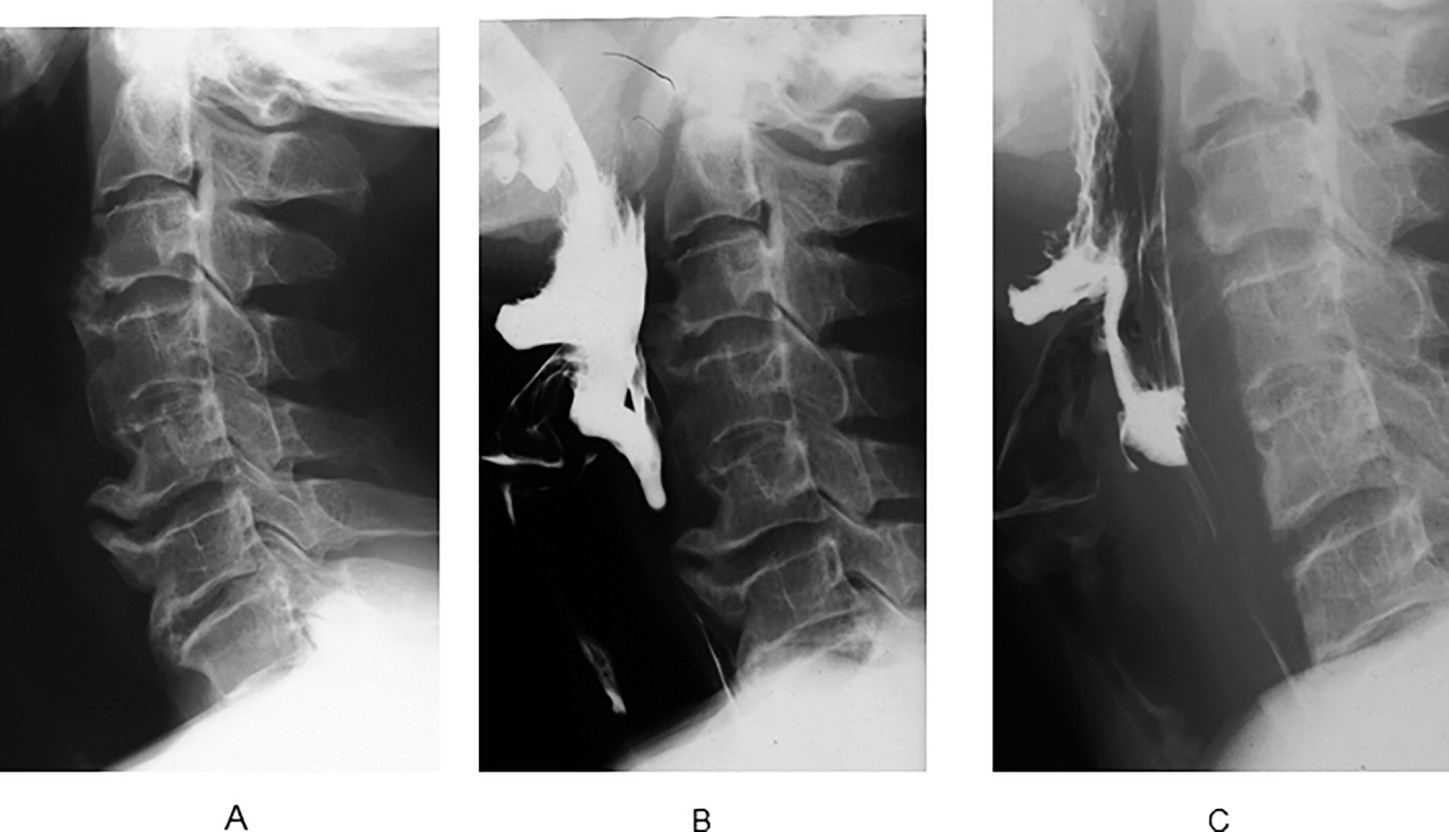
Preoperative and postoperative lateral radiographs of patient no. 4. (A) Cervical osteophytes at C5/6 and OPLL at C2/3. (B) Preoperative VFSS showed that the contrast medium was obstructed at C5/6 level and the esophagus was compressed. (C) Postoperative VFSS contrast medium was not obstructed, and the osteophyte at C5/6 was excised completely.
